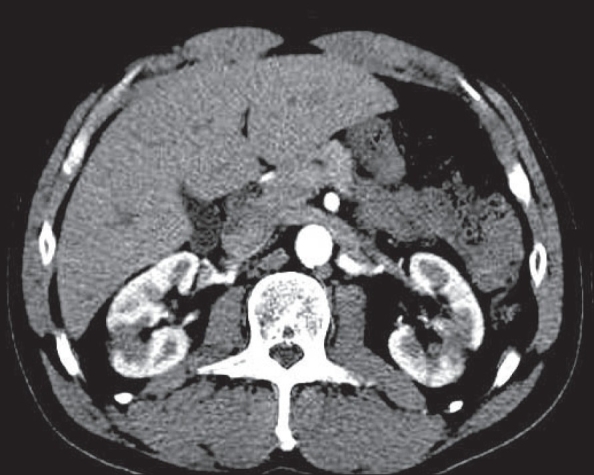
Acute pyelonephritis. Contrast-enhanced CT scan shows enlarged bilateral kidneys with streaky hypodensities (arrows) and minimal perinephric fat stranding
